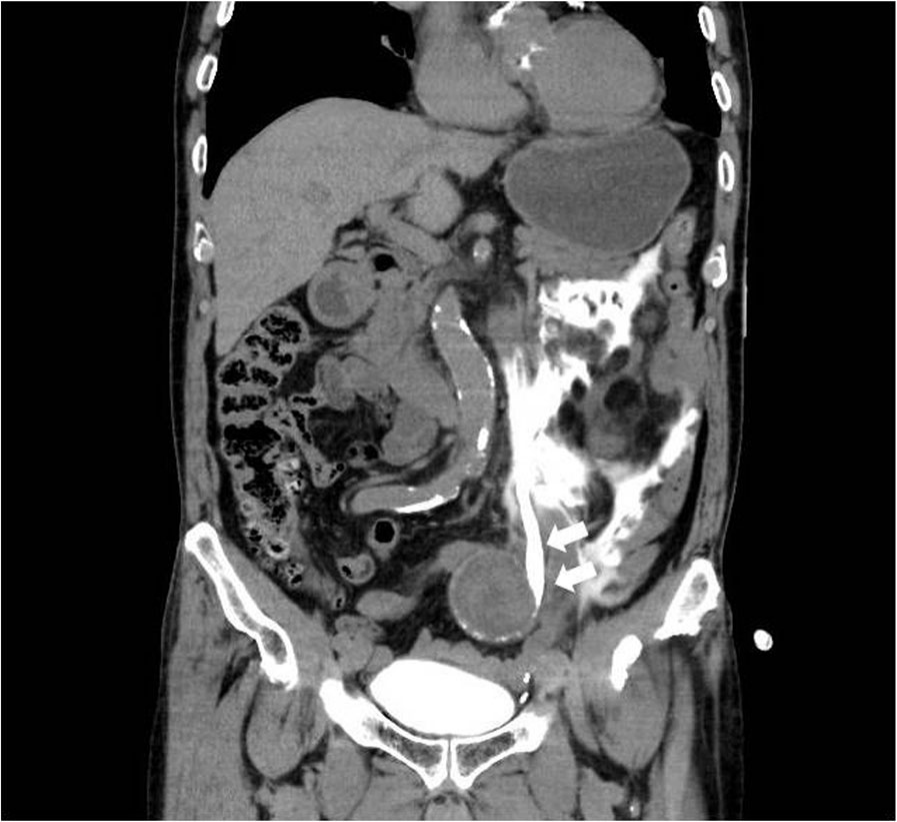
Computed tomography demonstrates a left hydroureteronephrosis and leakage of iodinated urine in the left-side retroperitoneum. Arrows indicate the left ureter compressed by a large iliac aneurysm
